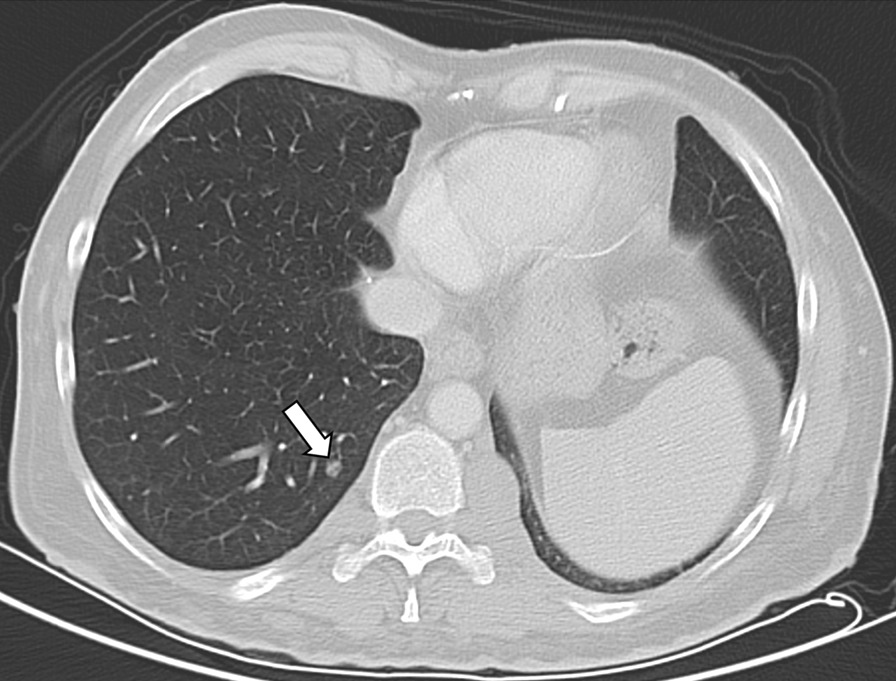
Transversal CT image of a 48-year old male non-smoker with a pulmonary nodule in the right lower lobe (white arrow)
